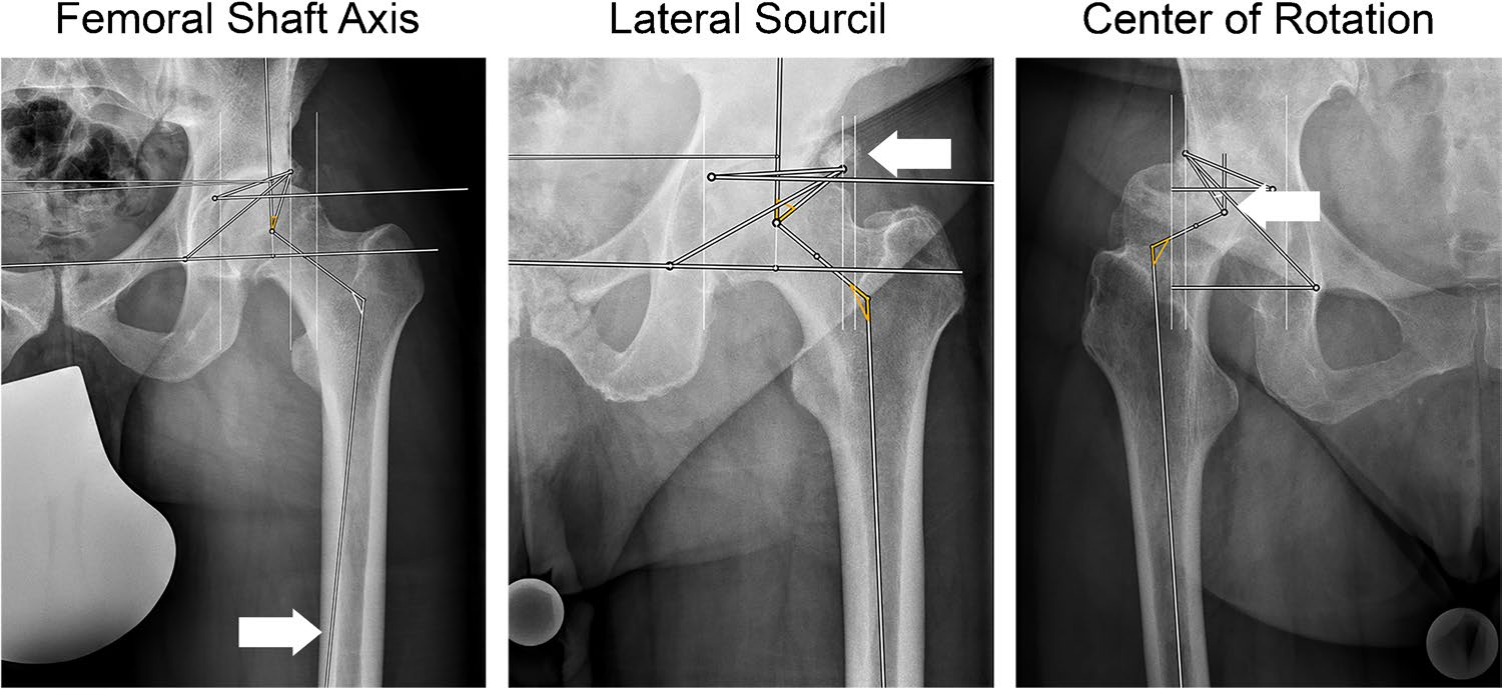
Example radiographs of erroneous landmark setting. Left, failed femoral shaft axis detection; middle, the lateral sourcil was erroneously set at an osteophyte; right, failed detection of the center of rotation and the femoral neck
